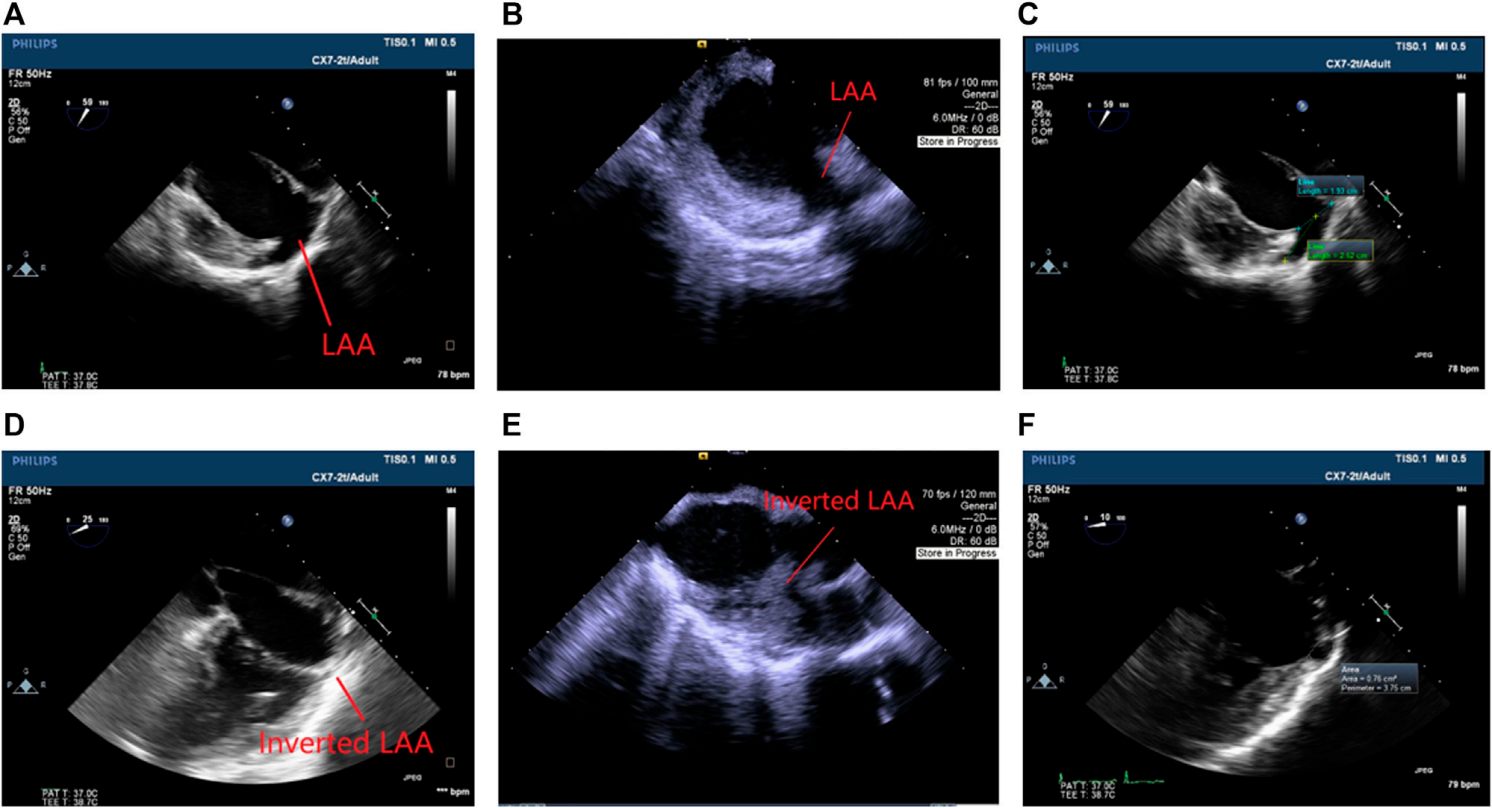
Echocardiogram images. (A) TEE before the procedure. (B) ICE before the procedure. (C) An example of LAA measurement before the procedure (blue: orifice diameter; green: depth). (D) TEE at 8 weeks after the procedure. (E) ICE at 8 weeks after the procedure. (F) An example of LAA measurement at termination (white ring: area). The inversion significantly reduced or eliminated the LAA space.
